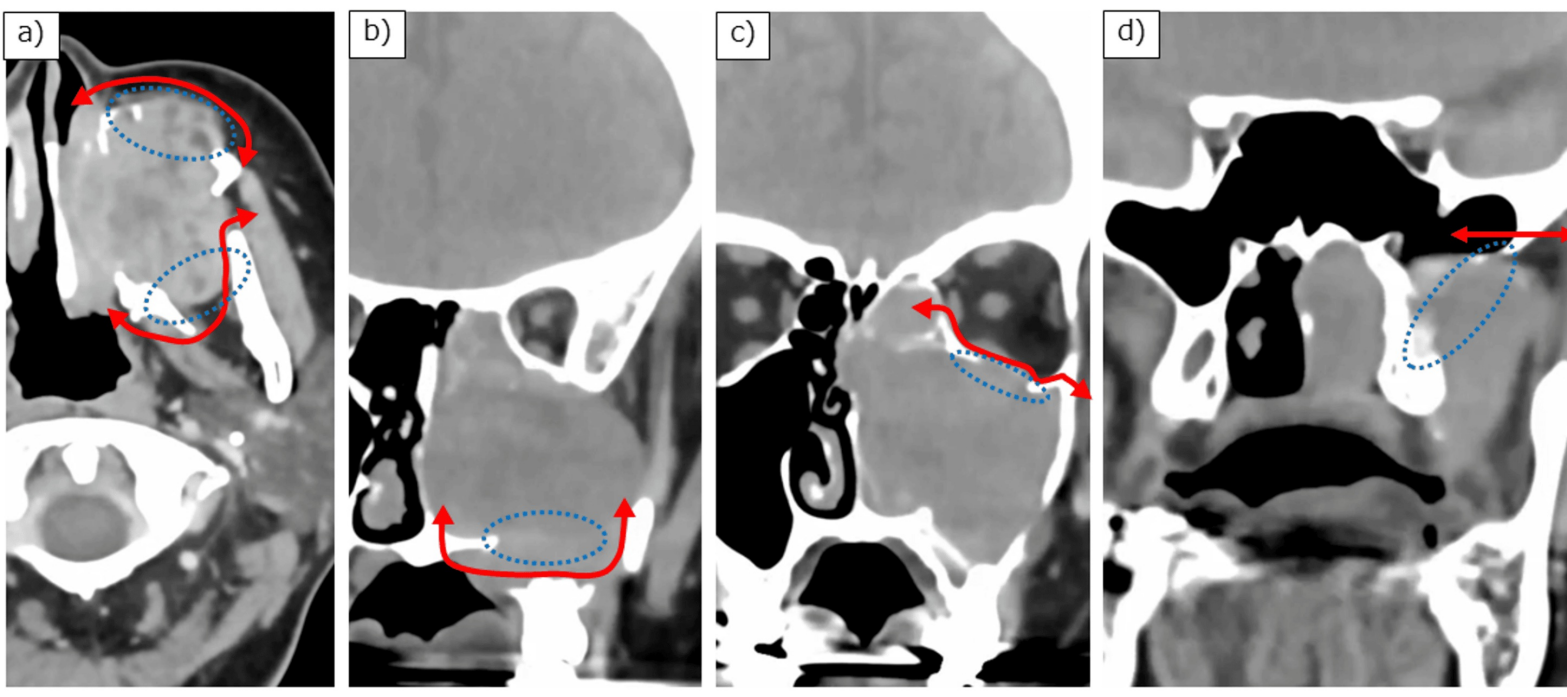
Preoperative computed tomography images. (a) Axial image showing bone infiltration in the anterior and posterior maxillary walls; (b, c) Coronal images showing infiltration in the inferior and superior maxillary walls, respectively; and (d) The base of the sphenoid pterygoid process. Blue dotted line: bone infiltration site; red arrow: resection line.
